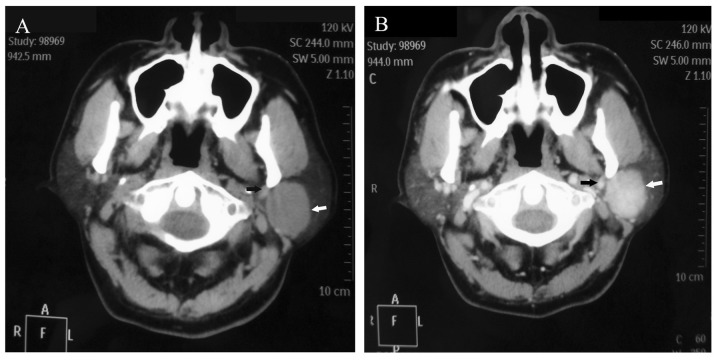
Imaging examination of the angioleiomyoma in the left parotid gland. (A) Computed tomography scan showing an ovoid lesion with a regular margin in the left parotid gland (white arrow), displacing the posterior facial vein (black arrow). (B) Enhanced computed tomography scan showing an ovoid, high-density lesion with heterogeneous enhancement (white arrow), together with enhancement of the posterior facial vein (black arrow).
